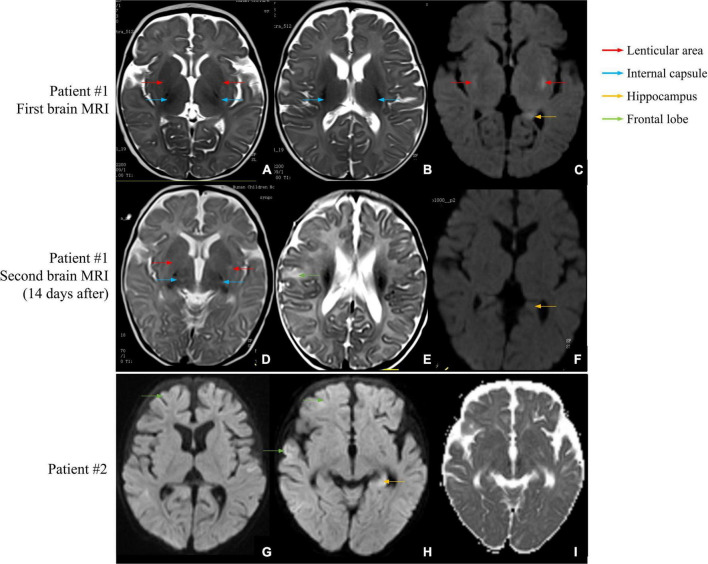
Brain MRI scans of patients #1 and #2. (A–C) First brain MRI examination of patient #1. (A,B) The T2-weighted image shows a small and slightly long T2 signal shadow in bilateral internal capsules and lenticular nuclei. (C) The diffusion-weighted image shows small patches of a slightly long T2 signal shadow in the upper outer capsule and left hippocampus. (D–F) Second brain MRI examination of patient #1. (D) The T2-weighted image shows a wider T2 signal shadow in bilateral internal and external capsules with higher signal intensity. (E) The T2 signal intensities are higher in the right frontal cortex and subcortical area than in the front. (F) No obvious abnormal signal is found in bilateral hippocampal regions, and no diffusion limitation is found in the lesions. (G–I) Brain MRI examination of patient #2. (G) The diffusion-weighted image shows wider and deeper sulcus fissures in bilateral cerebral hemispheres and slightly higher cortical signal in part of the cerebral hemispheres. (H) Partial cortex of the cerebral hemisphere and the left hippocampus show slightly high signal intensities. (I) The apparent diffusion coefficient map shows a slightly low signal intensity, indicating limited dispersion.
